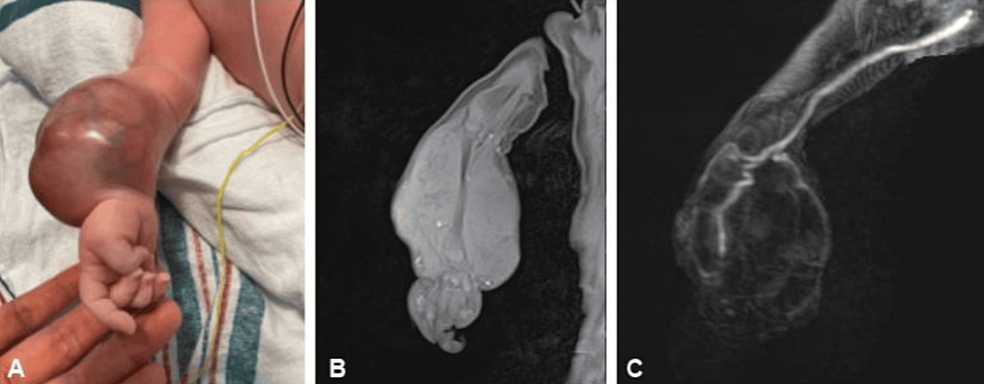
Soft tissue mass on the right forearm (A) that underwent T1-weighted MRI (B) and MRA (C) MRI: magnetic resonance imaging; MRA: magnetic resonance angiogram
